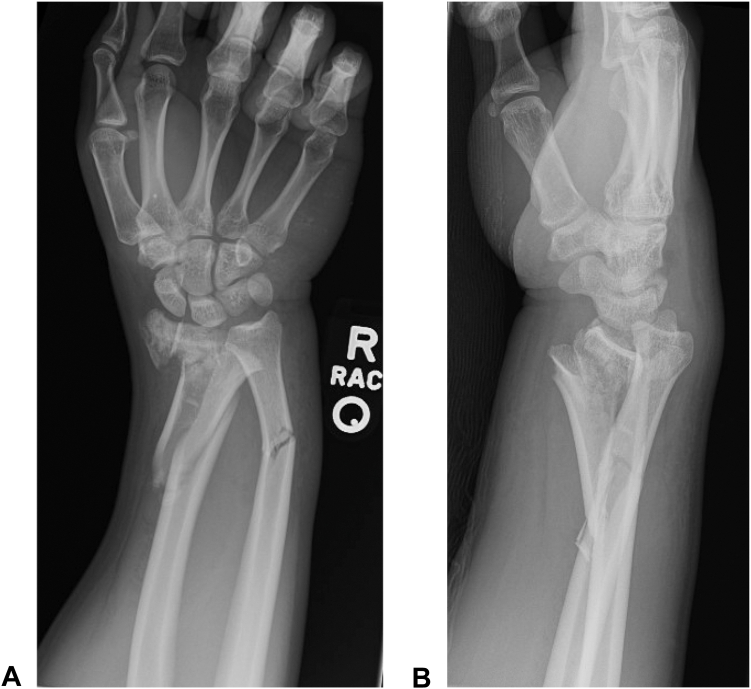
Example of wrist and forearm injury sustained from fall from border wall. A Anterior-posterior and B lateral x-rays demonstrate an example of an upper-extremity injury with distal fracture of both the radius and the ulna that can be sustained from a fall from significant height.
